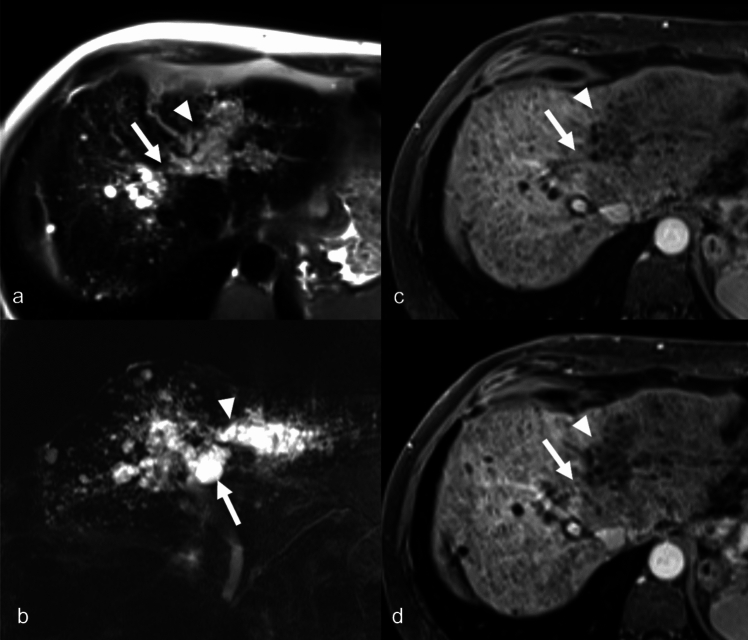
Peribiliary cysts mimicking cholangiocarcinoma in a 54-year-old female with history of hepatitis C, alcoholic cirrhosis, and chronically elevated total bilirubin (4.3 mg/dL) presenting with concern for a suspicious left medial lobe liver lesion on a pre-transplant CT chest with contrast. Axial T2-weighted image (a) demonstrates a cirrhotic liver that appears dark due to iron deposition. Cystic lesions are visualized along the right and left (arrowhead) ducts extending from the hilum (arrow). The cystic conglomerate in the left lobe was the area of concern on prior CT. On thick-slab MRCP (b), the lesions appear more round rather than as dilated ducts consistent with peribiliary cysts. Late arterial phase (c) and delayed phase (d) MRI shows the cystic region in the left hepatic lobe (arrowhead) and the hilar region (arrow) with no abnormal enhancement to suggest underlying mass. CA-19 was normal and brushings by ERCP were negative
